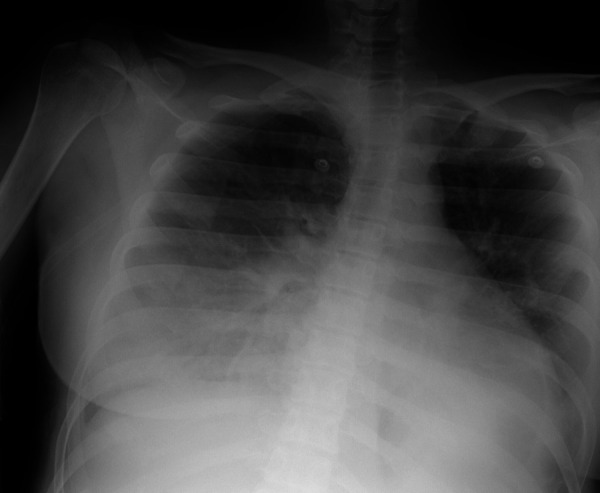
Chest X-ray of the patient showing bilateral lower lobe consolidation with pleural effusion.
